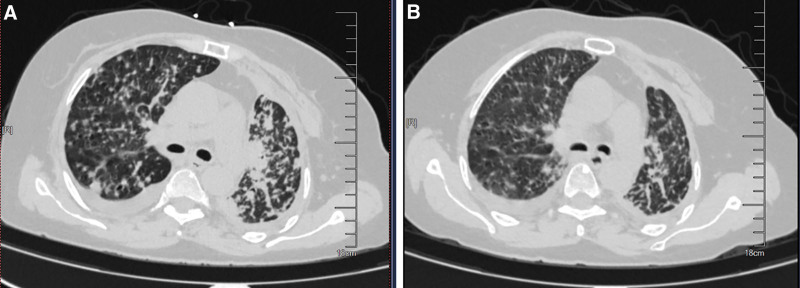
The temporal progression of lorlatinib resistance and the subsequent therapeutic response to cabozantinib were evaluated over a 4-mo period. (A) Initial axial CT imaging performed on September 20, 2023, demonstrated the presence of bilateral pulmonary metastatic lesions, consistent with disease progression and the development of acquired resistance to lorlatinib. (B) Follow-up axial CT imaging conducted on February 2, 2024, after 4 mo of cabozantinib treatment, revealed a marked reduction in both the size and density of multiple metastatic nodules within the bilateral lung fields, with only minimal residual bilateral pleural effusion observed. CT = computed tomography.
